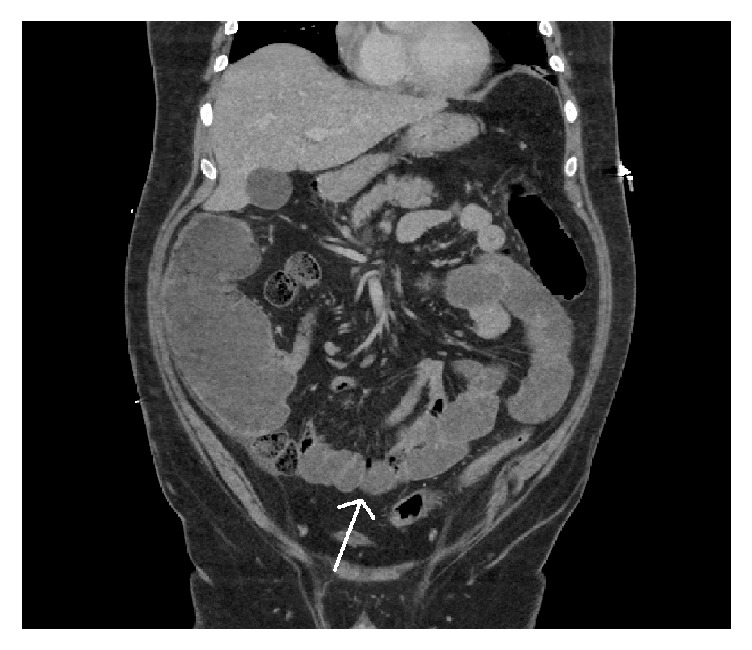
Coronal view from CT enterography demonstrating narrowing of the distal and terminal ileum as well as a short segment of narrowing in the mid ileum (arrow) with fecalization in the distal ileum.
